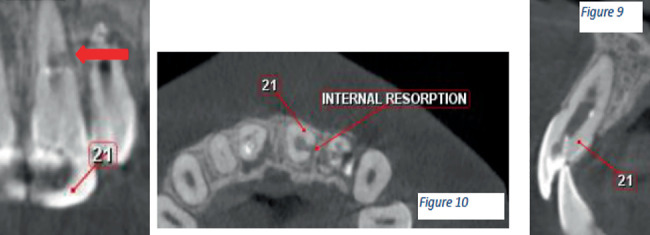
CBCT images showing coronal, axial and sagittal views of Tooth Number 21 with internal root resorption.
